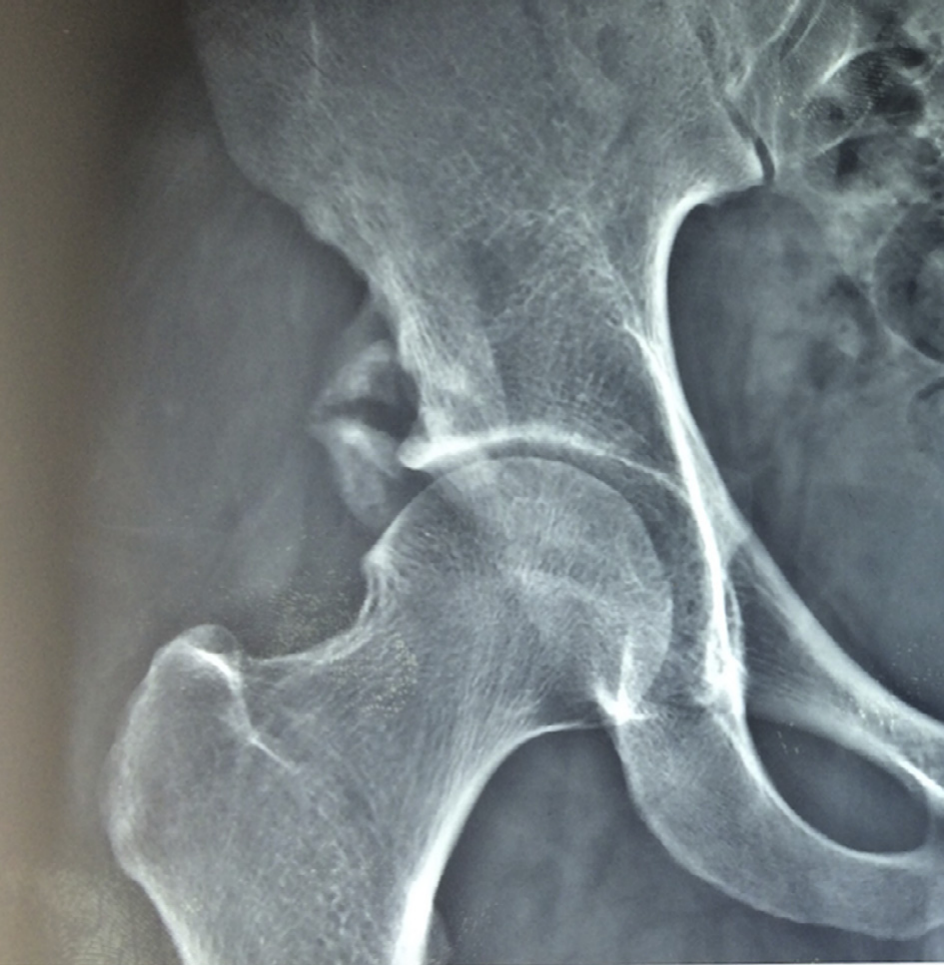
X-ray of right hip showing avulsion fracture of anterior inferior iliac spine in a young footballer.
